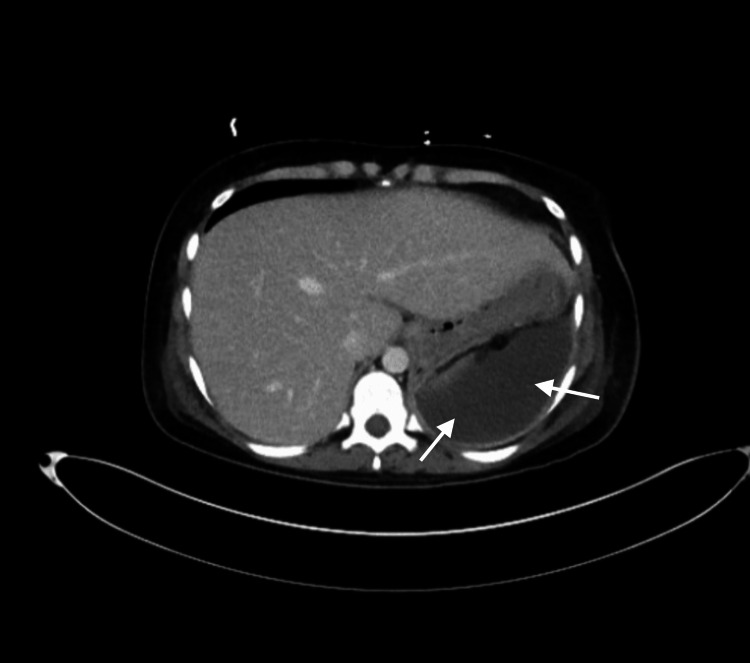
Transverse CT image of the abdomen prior to laparotomy showing perisplenic fluid collection CT, computed tomography.
